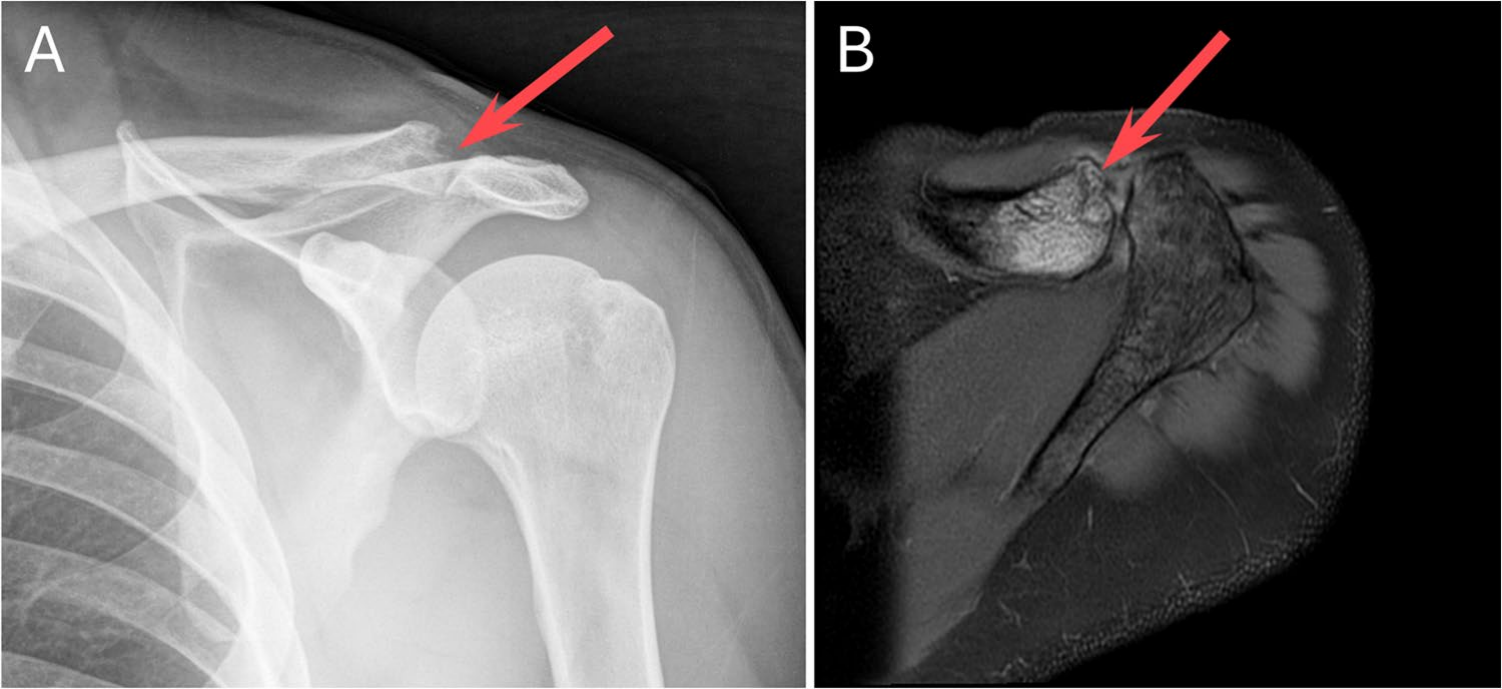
A Externally rotated AP shoulder radiograph demonstrates classic X-ray findings of DCO, with osseous resorption of the distal clavicle (arrow). B Axial PD fat-suppressed MR image of the shoulder demonstrates classic MRI findings of DCO, including intense bone marrow edema and osseous resorption of the distal clavicle (arrow)
